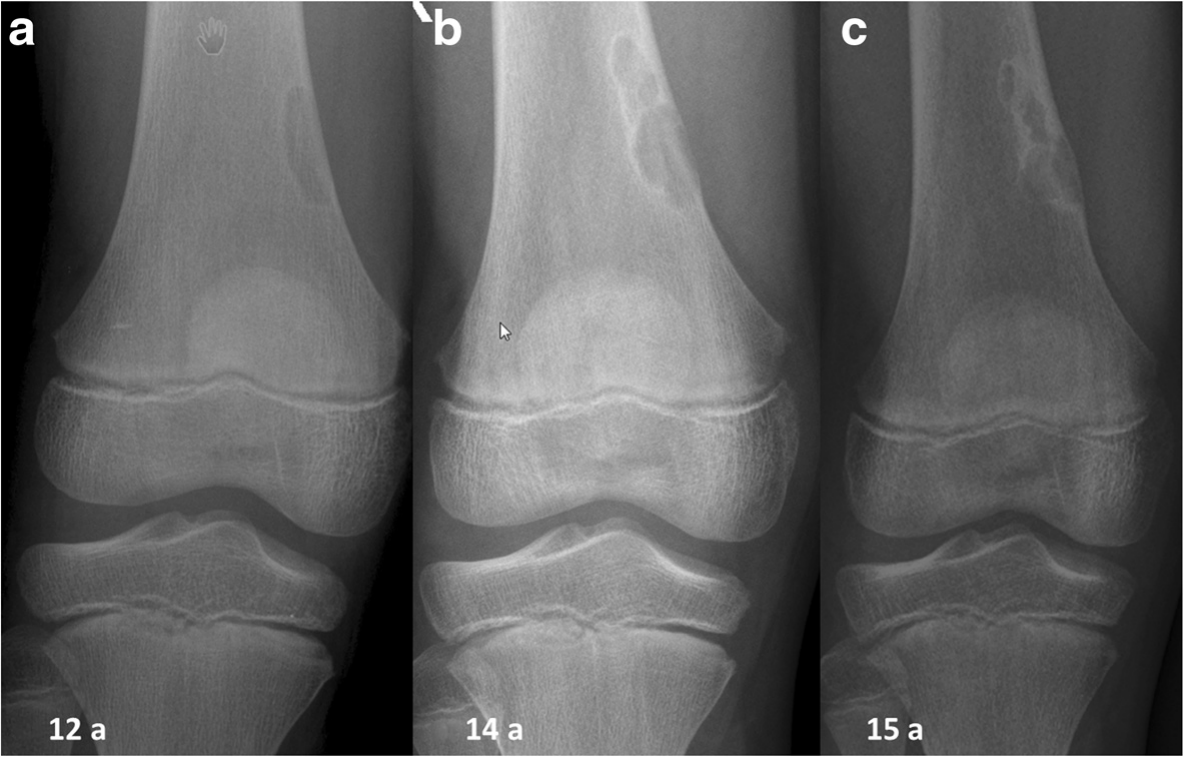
(a) A boy at the age of 12 years at first presentation. Anteroposterior radiographs showing a lytic lesion of the right distal femur representing a stage A lesion. (b) 2 years later the lesion was polycyclic in shape with clearly sclerotic borders (stage B). (c) At the age of 15 years there was evidence of ossification beginning at the diaphysis (stage c)
